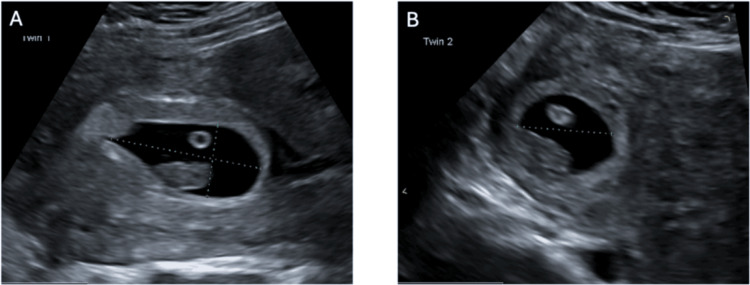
Transvaginal ultrasound demonstrating a heterotopic pregnancy (A) Intrauterine pregnancy with a gestational sac corresponding to approximately 7+5 weeks gestation. (B) Cornual ectopic pregnancy with a gestational sac corresponding to approximately 7+5 weeks gestation.
